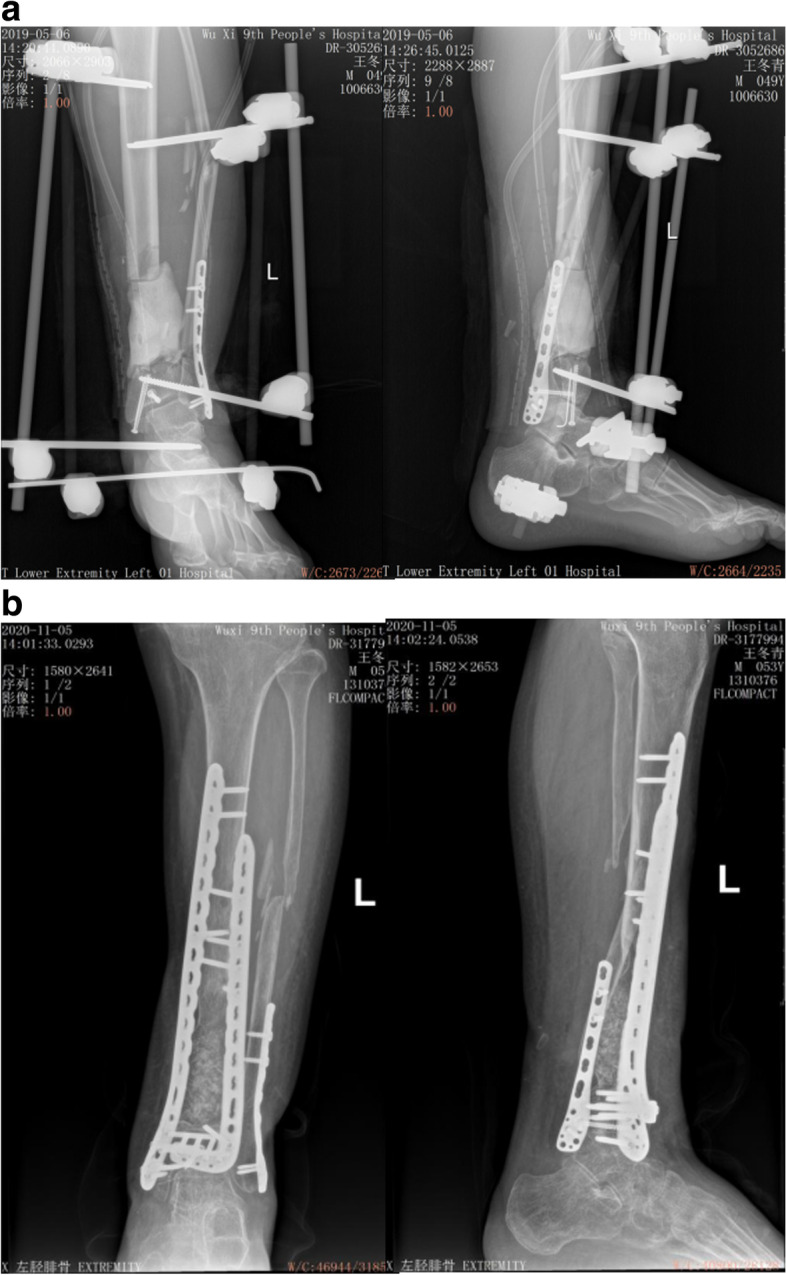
A 49 years-old male patient with segmental defects of the left tibia treated with IMT. a The cement spacer was prepared by extracorporeal formation. B X-rays showed the defects clinically healed in 7 months
